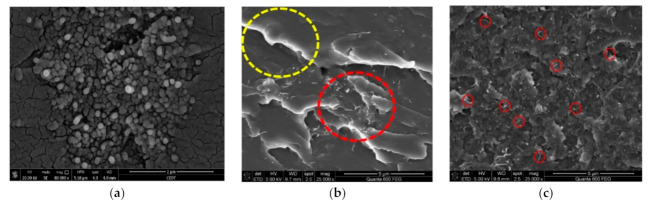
SEM image of (a) agglomoration of unmodified MWCNTs (0.5 wt/wt.%), (b) nonhomogenous dispersion of unmodified MWCNTs in epoxy resin, and (c) good dispersion of F-MWCNTs using ultrasonic mixing technique.
